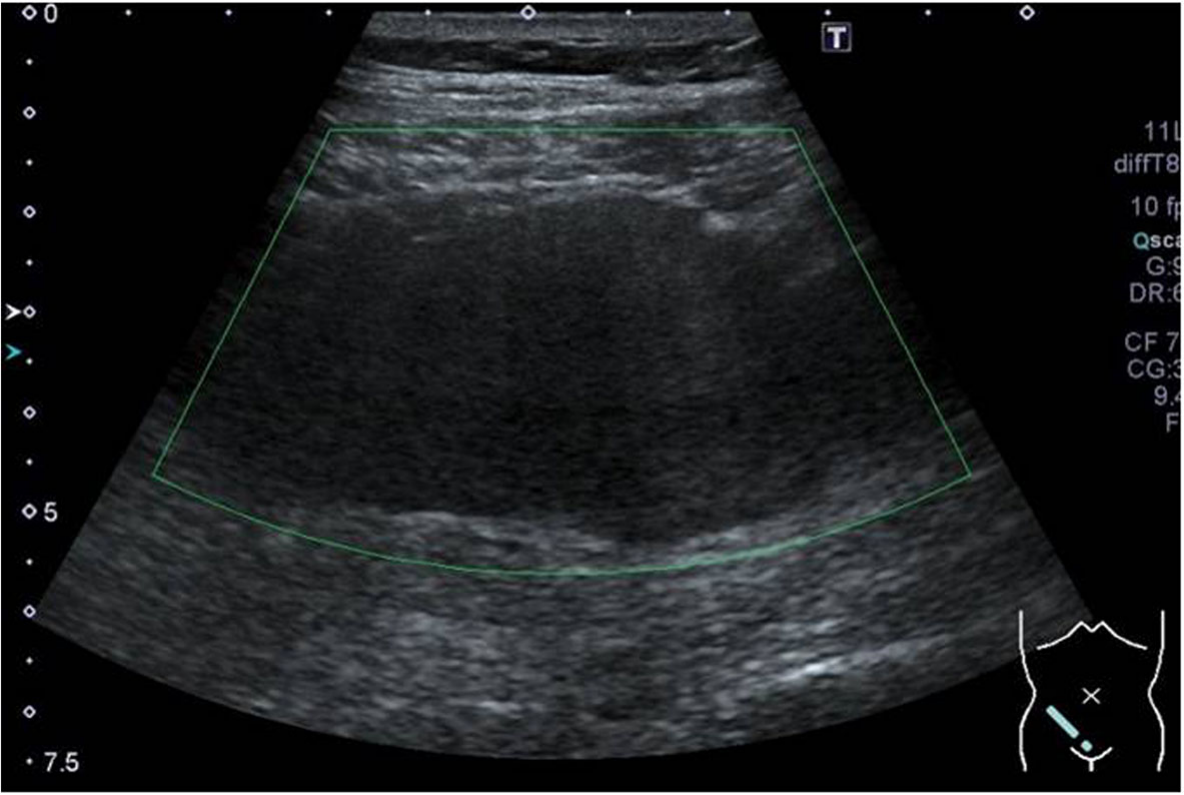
Transabdominal ultrasonographic image of a tumor measuring 10 cm in diameter showing a homogeneous echo pattern
